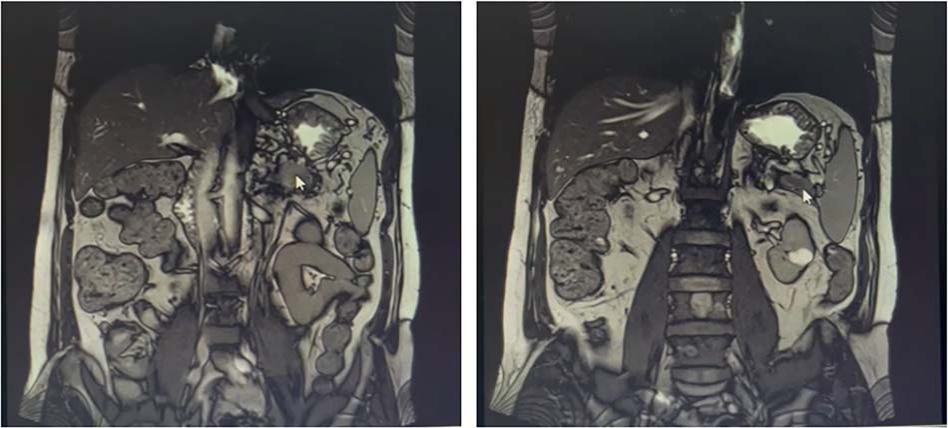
Metastatic masses can be seen in the pancreatic tissue on the CT-Scan (coronal view)
